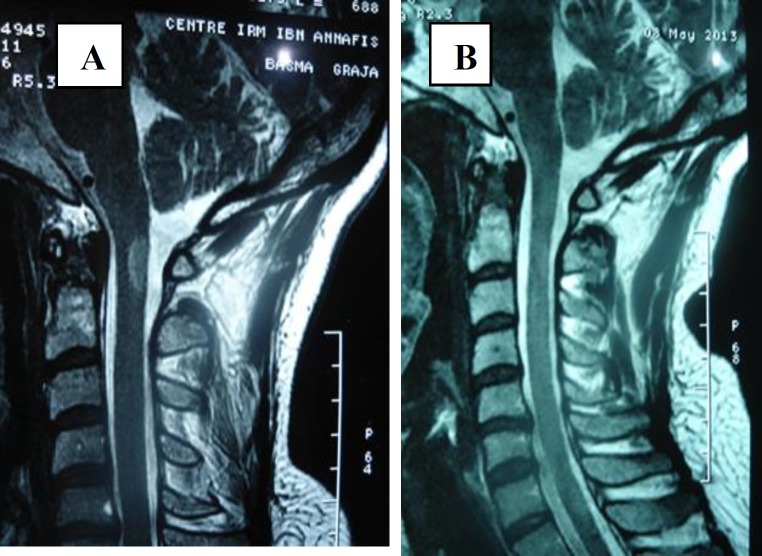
Cerebral and spinal MRI demonstrated isointensities on T1 weighted images, T2 hyperintensities extending from the 3^rd^ to the 9^th^ thoracic vertebrae with a strong enhancing Gadolinium signal
